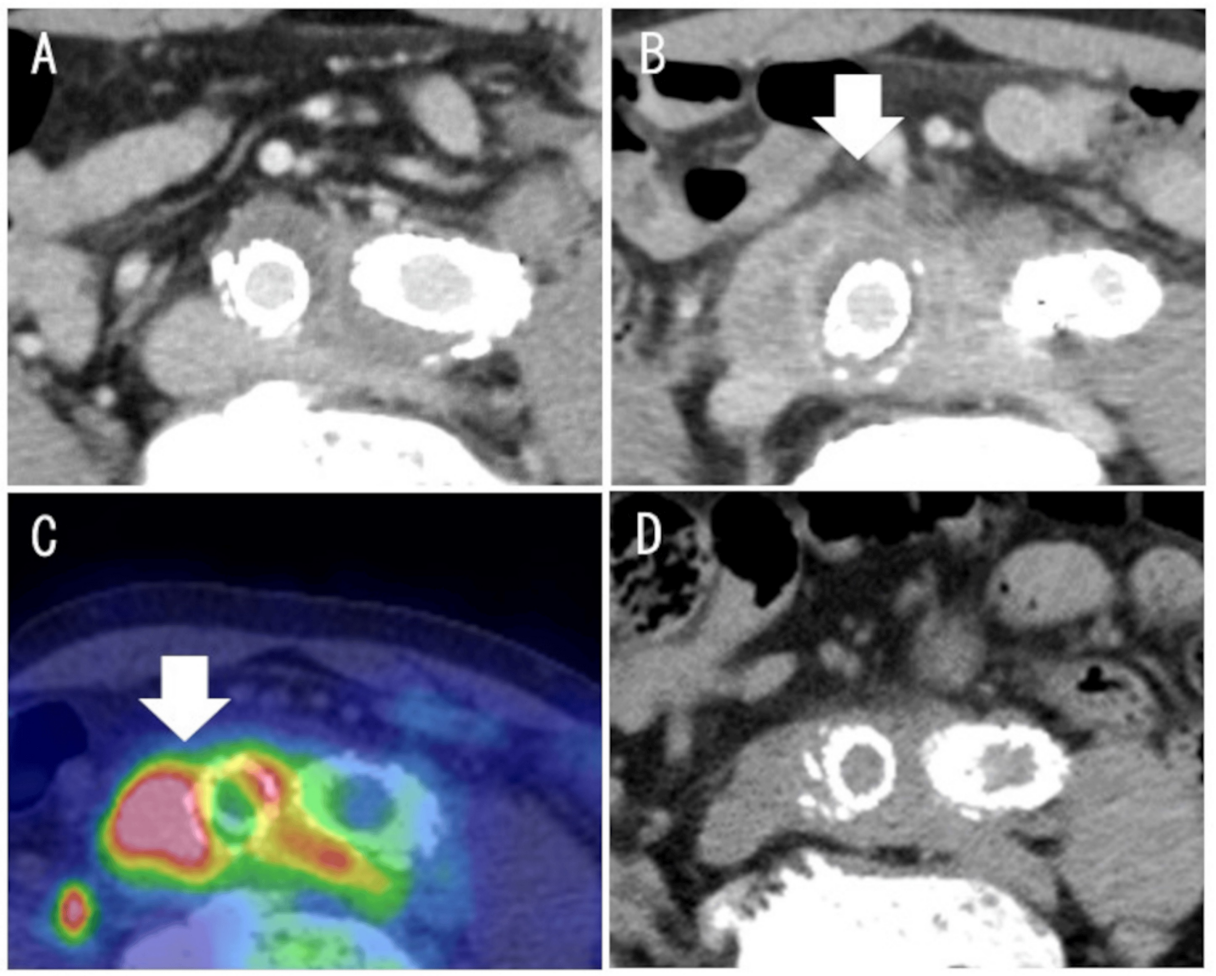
Chronological change in CT images after endovascular aneurysm repair Periarterial thickening was not observed on CT on postoperative day 5 (A); however, it was observed at postoperative month 2 (B), along with steroid reduction. (C) 18F-fluorodeoxyglucose positron emission tomography-CT performed at postoperative month 2 demonstrated increased metabolic activity corresponding to the area of periarterial thickening, with a standardized uptake value of 11.56. (D) Periarterial thickening decreased with steroid therapy at postoperative month 4. White arrows indicate periarterial thickening. CT: computed tomography
